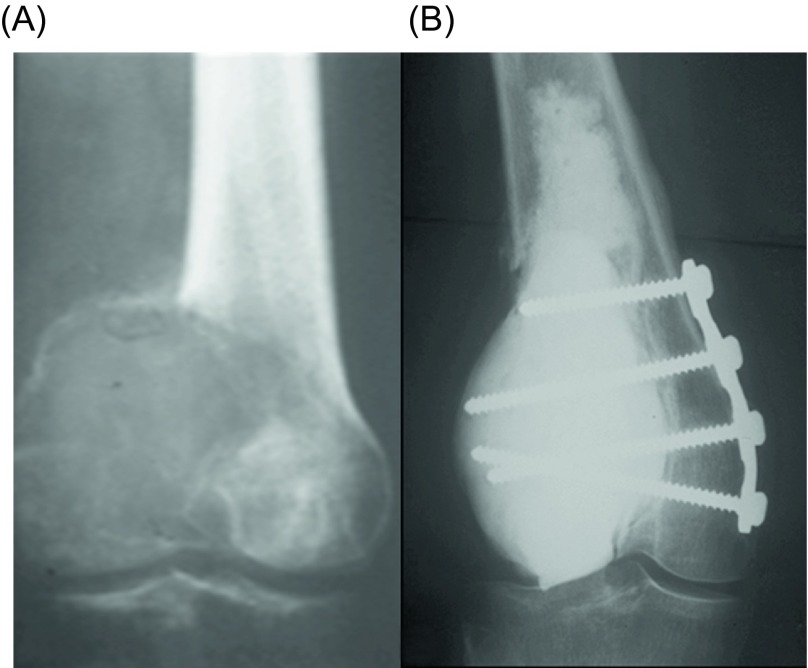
(A) Anteroposterior radiograph of the left knee of a 46-year-old man with a GCT of the medial femoral condyle. (B) Anteroposterior radiograph of the left knee seven years after curettage, cauterization, and cementation, in addition to short plate osteosynthesis shows no evidence of local tumor recurrence.
